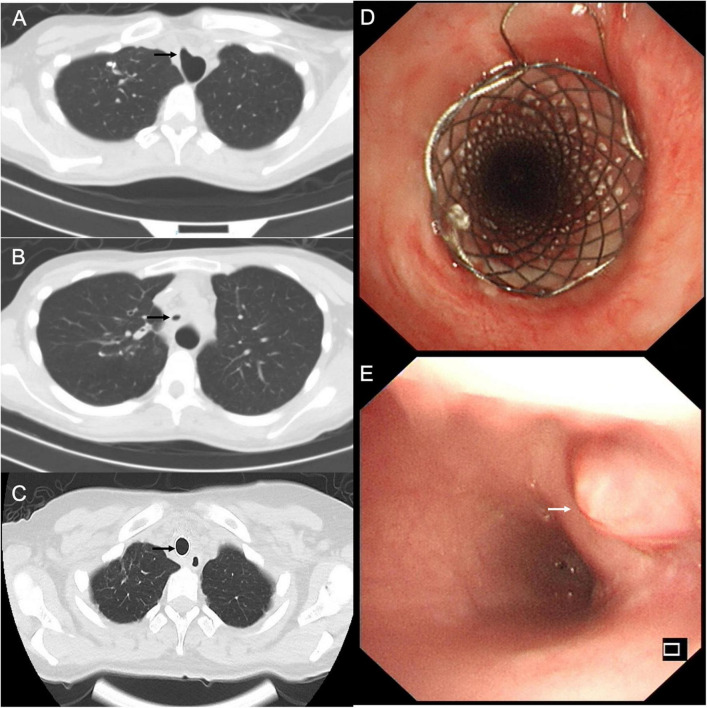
(A) The chest computed tomography (CT) showed the tracheoesophageal fistula (TEF) in the trachea (black arrow). (B) The chest CT performed after the stent removal demonstrated a diffuse tracheal stenosis, the narrowest part being 5.7 mm in diameter (black arrow). (C) Axial chest CT view confirmed the closure of TEF and patency of the trachea (black arrow). (D) The fully covered self-expandable metallic stent extended more than 10 mm beyond the TEF to occlude the orifice and establish the patency of the tracheobronchial tree. (E) The endoscopic view of TEF was fully covered by epithelium and granulation tissue (white arrow).
